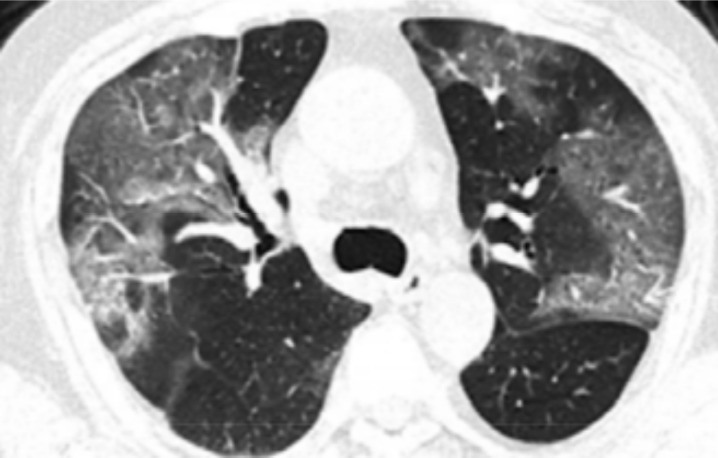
COVID-19 pneumonia: section CT shows bilateral GGO
